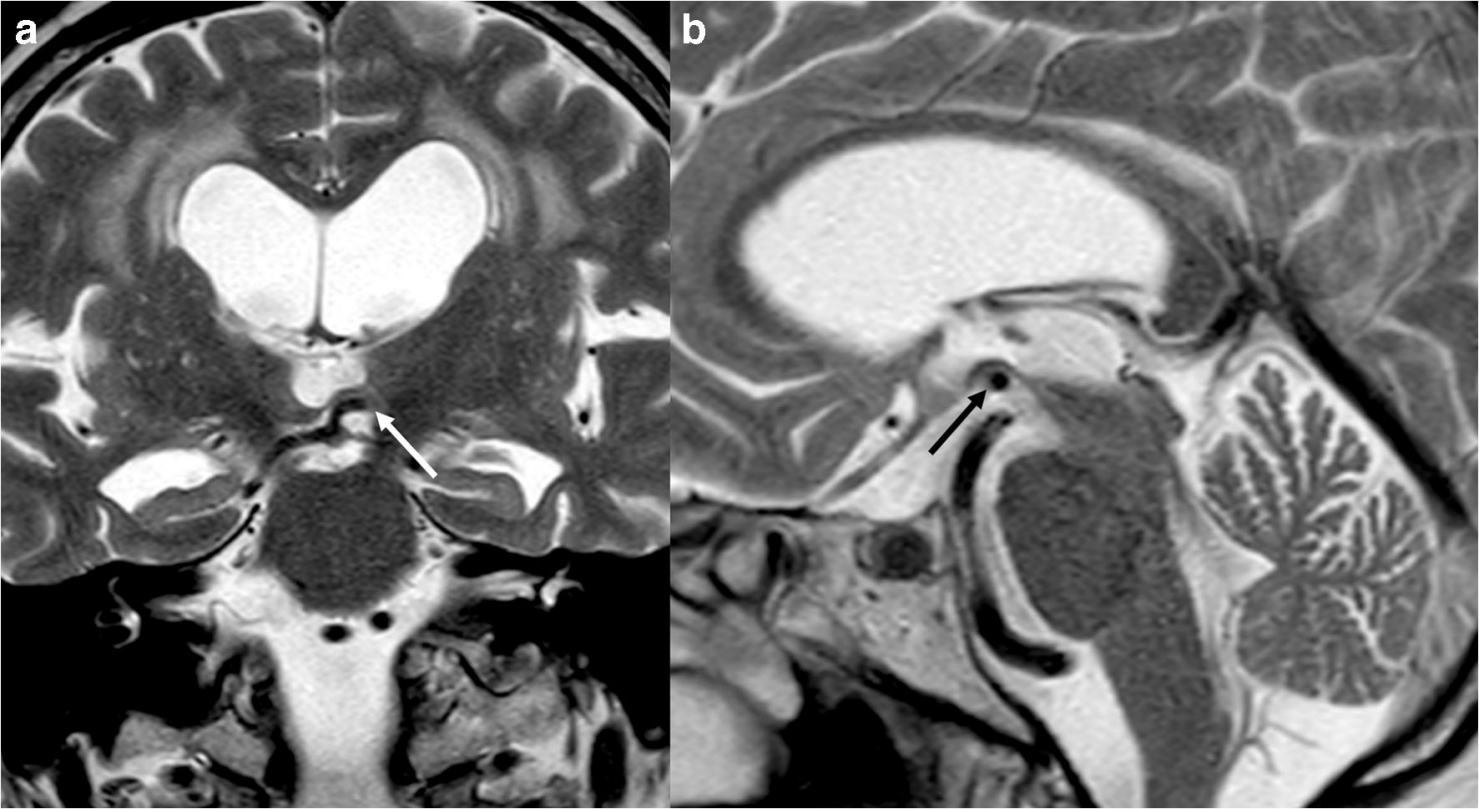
Coronal (a) and sagittal (b) T2-weighted images showing upper displacement of the third ventricle floor by the left posterior cerebral artery
